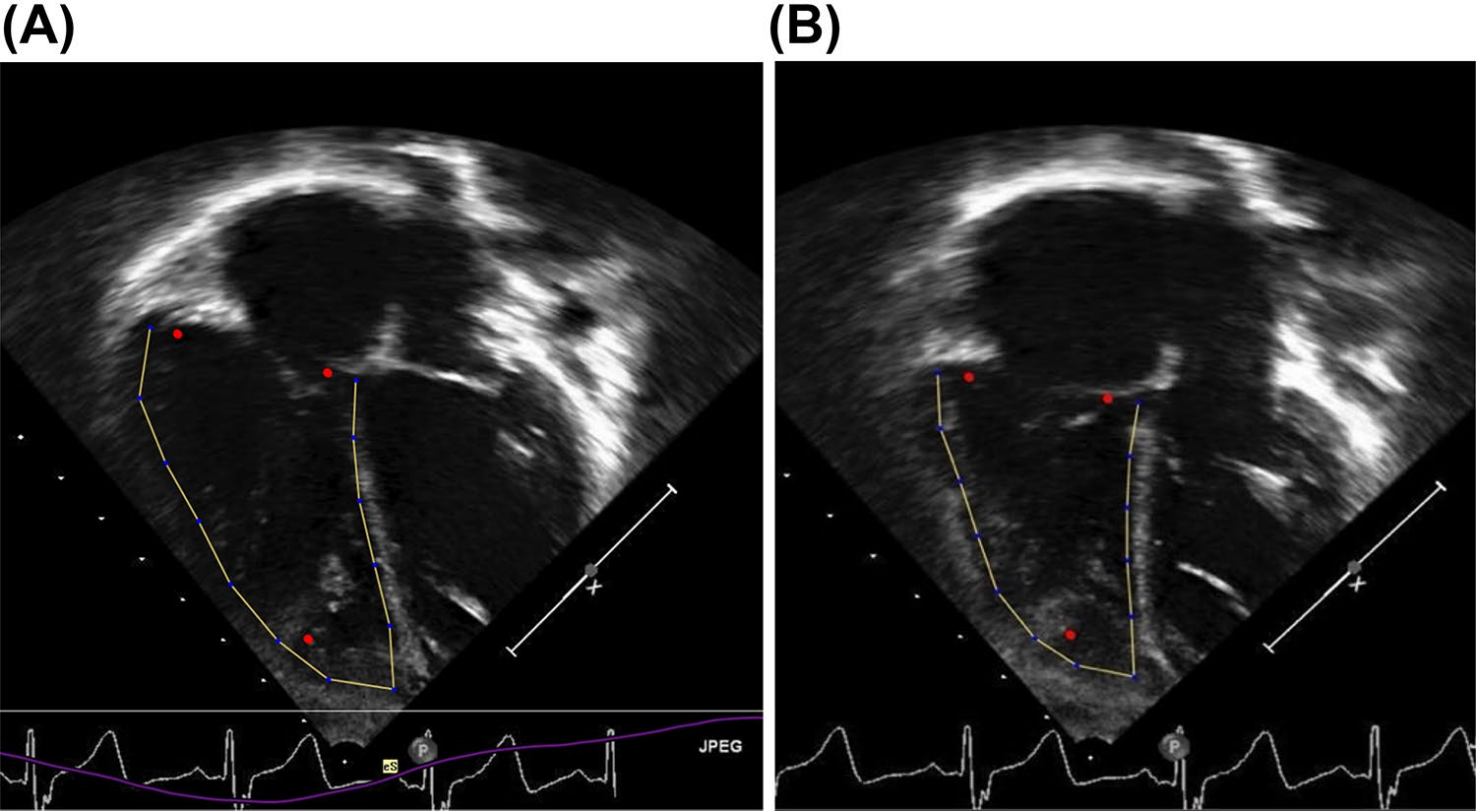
Echocardiographic measurements. Echocardiographic four-chamber view. The RV endocardium was traced at end-diastole (A) and end-systole (B) (Yellow boundaries)
